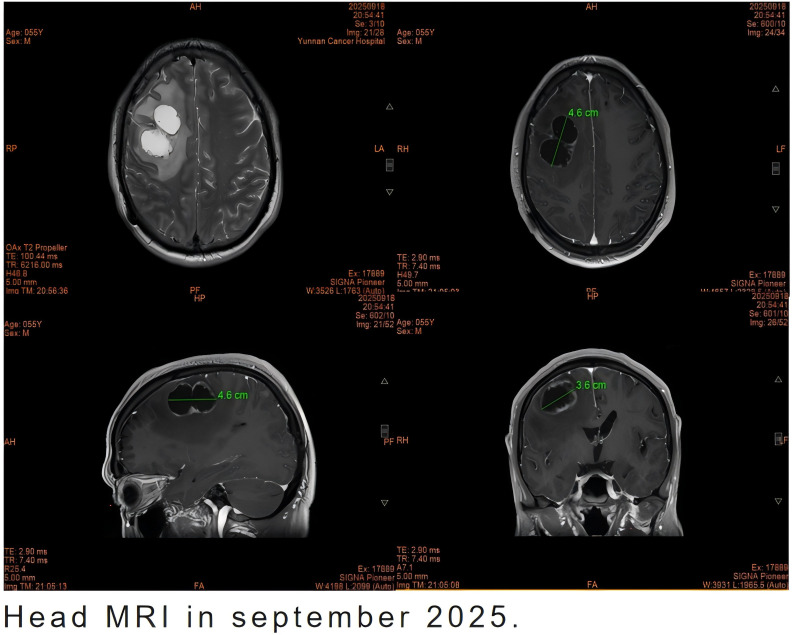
Head MRI in September 2025.
